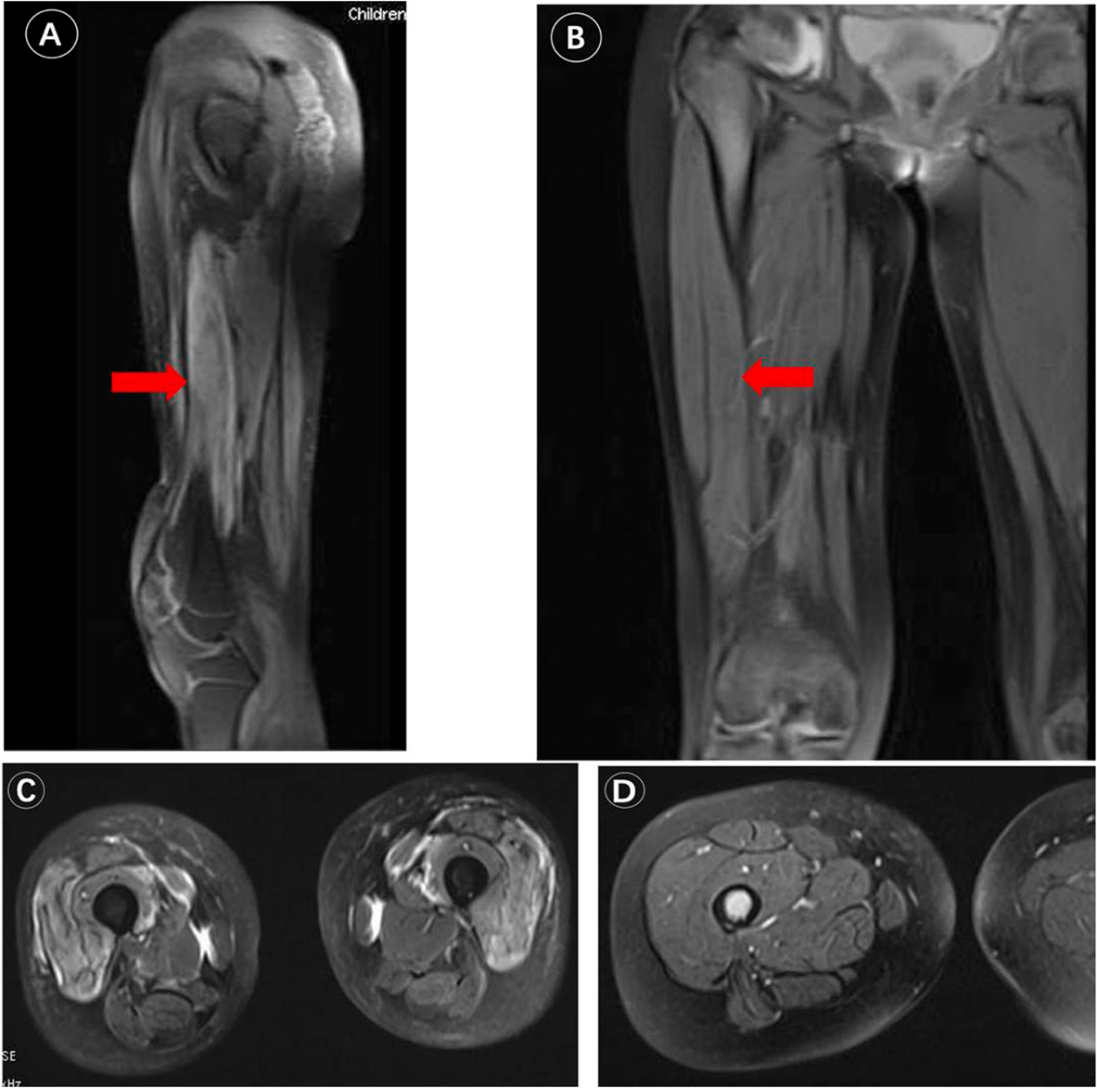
Magnetic resonance imaging of left lower limbs of patient 1. a and c revealed abnormal signals in muscle and fluid accumulation in the intermuscular space considering inflammatory changes. b and d revealed abnormal changes recovered after tocilizumab
